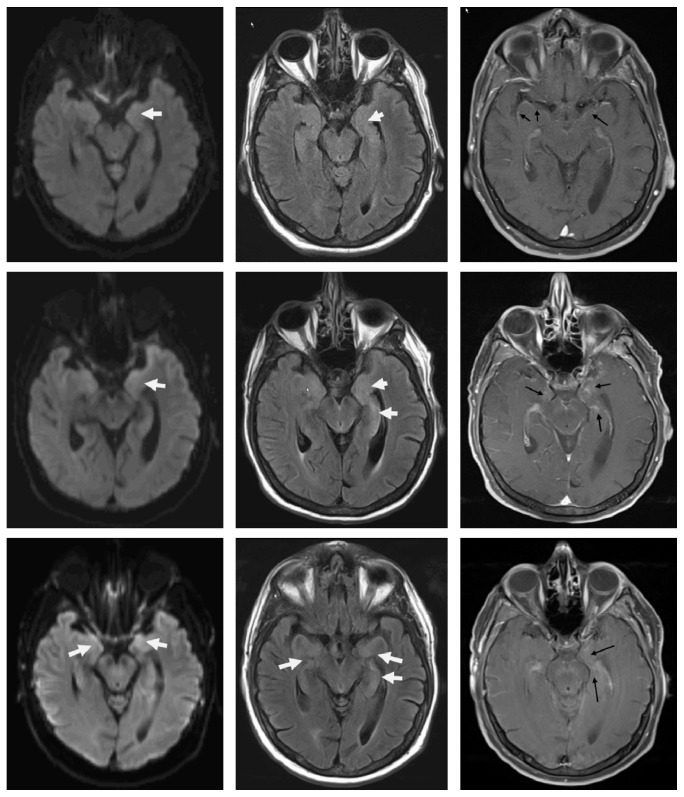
The first row shows brain MRI (from left to right, DWI, FLAIR, and T1+C) from first ER visit with presumed TGA: arrows show subtle signal changes that were too mild to be called. The middle row shows brain MRI (from left to right, DWI, FLAIR, and T1+C) six weeks from his first presentation: signal changes in DWI, FLAIR in hippocampus; moreover, there is enhancement in hippocampus and medial temporal lobe. The lowest row shows that brain MRI after treatment shows subtle improvement in hippocampus and temporal areas.
